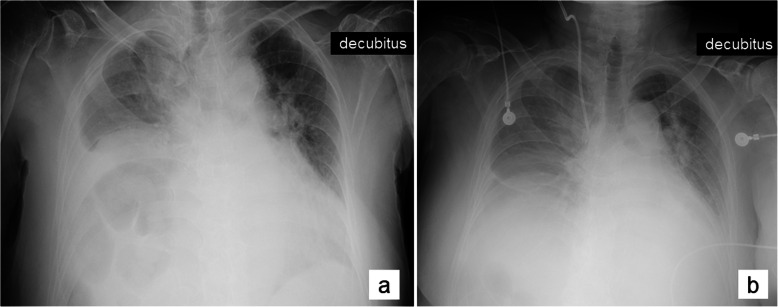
Chest radiographs a before surgery and b after extubation. a Elevation of the right hemidiaphragm, right-sided pleural effusion, and dilated bowel were shown. b An increase in the right lung volume was shown
